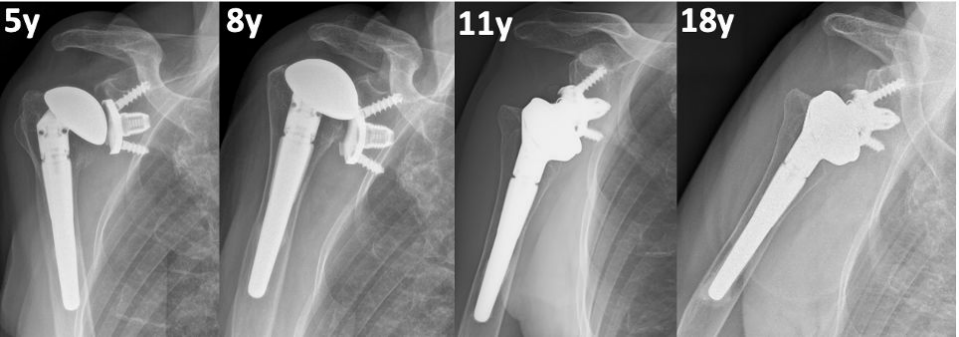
Anteroposterior radiograph views showing a 70-year old female patient who had a traumatic rotator cuff rupture after eight years and underwent conversion to reverse shoulder arthroplasty maintaining the original convertible metal-backed glenoid. After 18 years from the first surgery, the implant is still stable.
